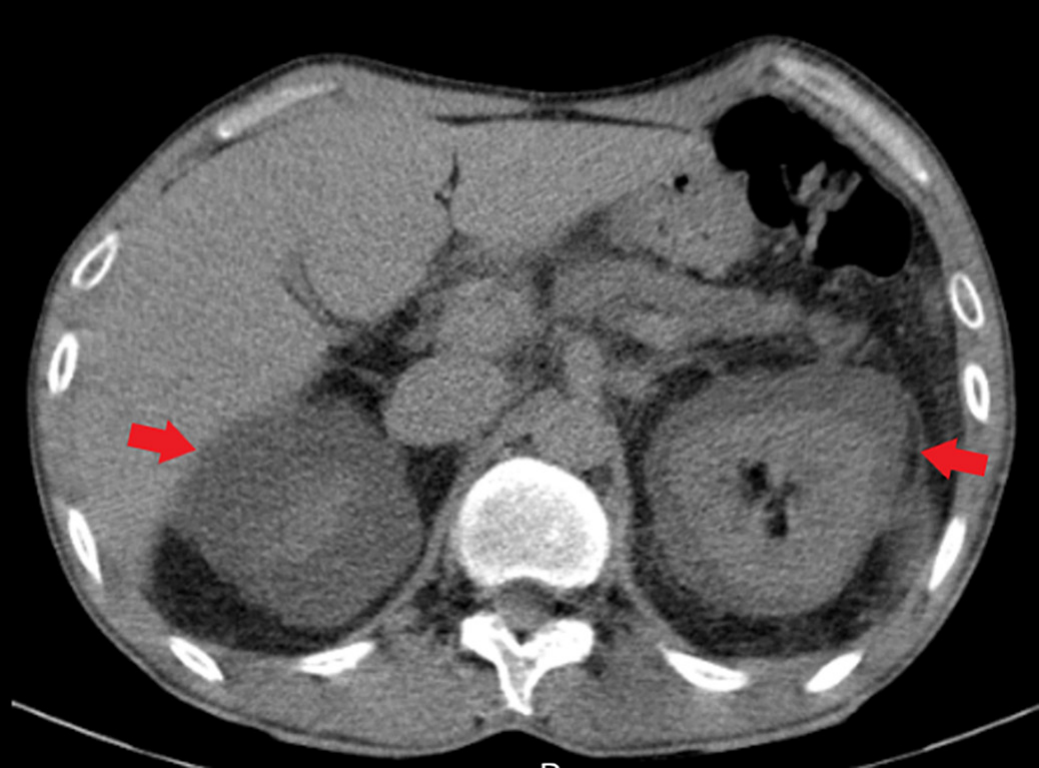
Non‐contrast abdominal CT scan. Axial image shows bilateral perirenal fluid accumulation (red arrows).
